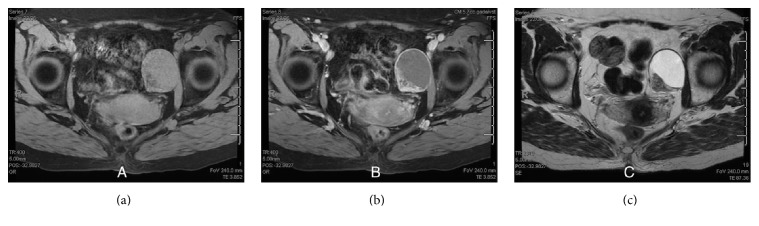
MRI of the pelvic mass with (a) T1, (b) T1 + contrast, and (c) T2 images.
